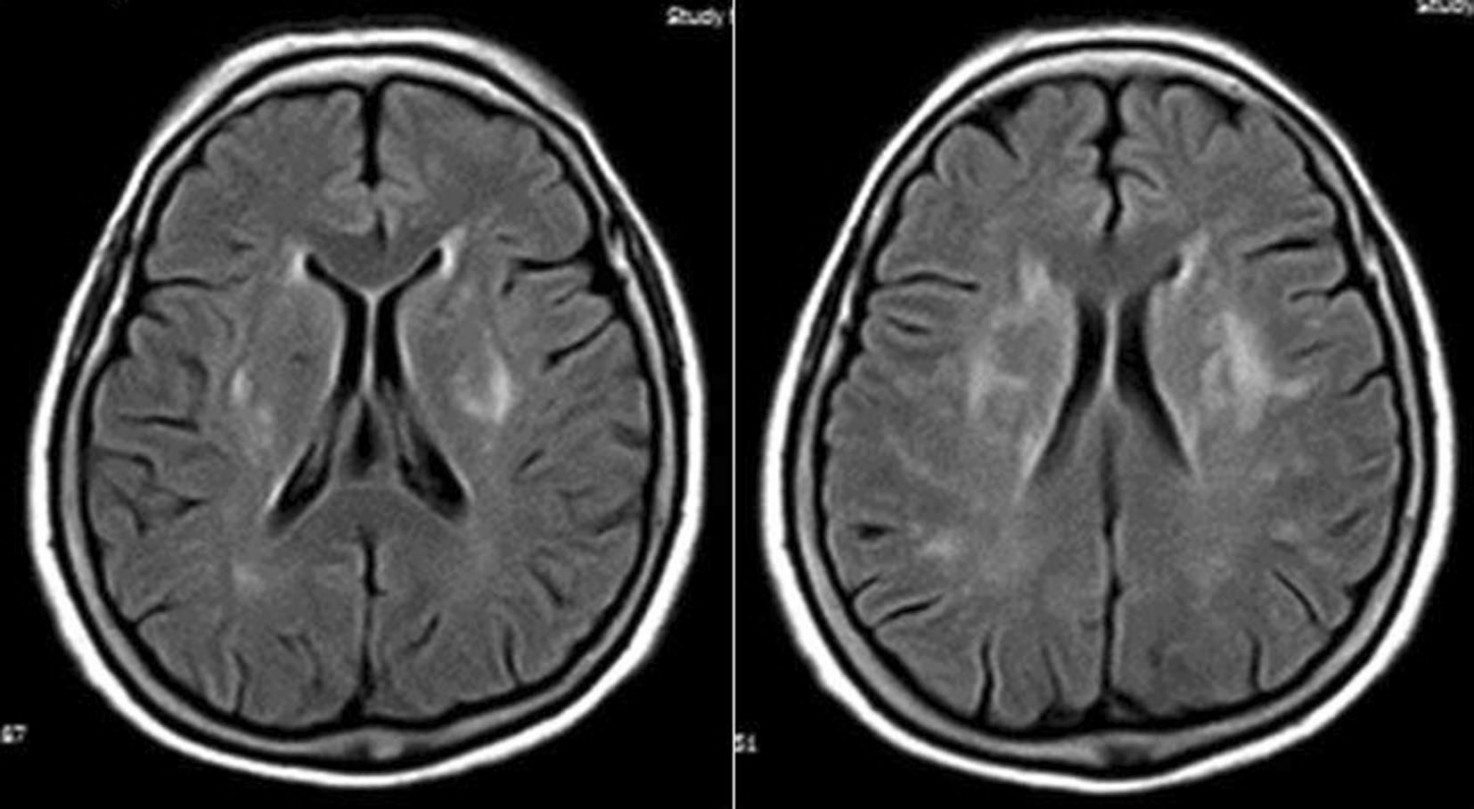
Fluid-attenuated inversion recovery magnetic resonance imaging (TR/TE/TI = 9000/120/2500 ms). Both images show severe white matter hyperintensities of presumed vascular origin according to the modified Fazekas scale. Of note is the extension of periventricular lesions into the subcortical white matter and large confluent subcortical foci
